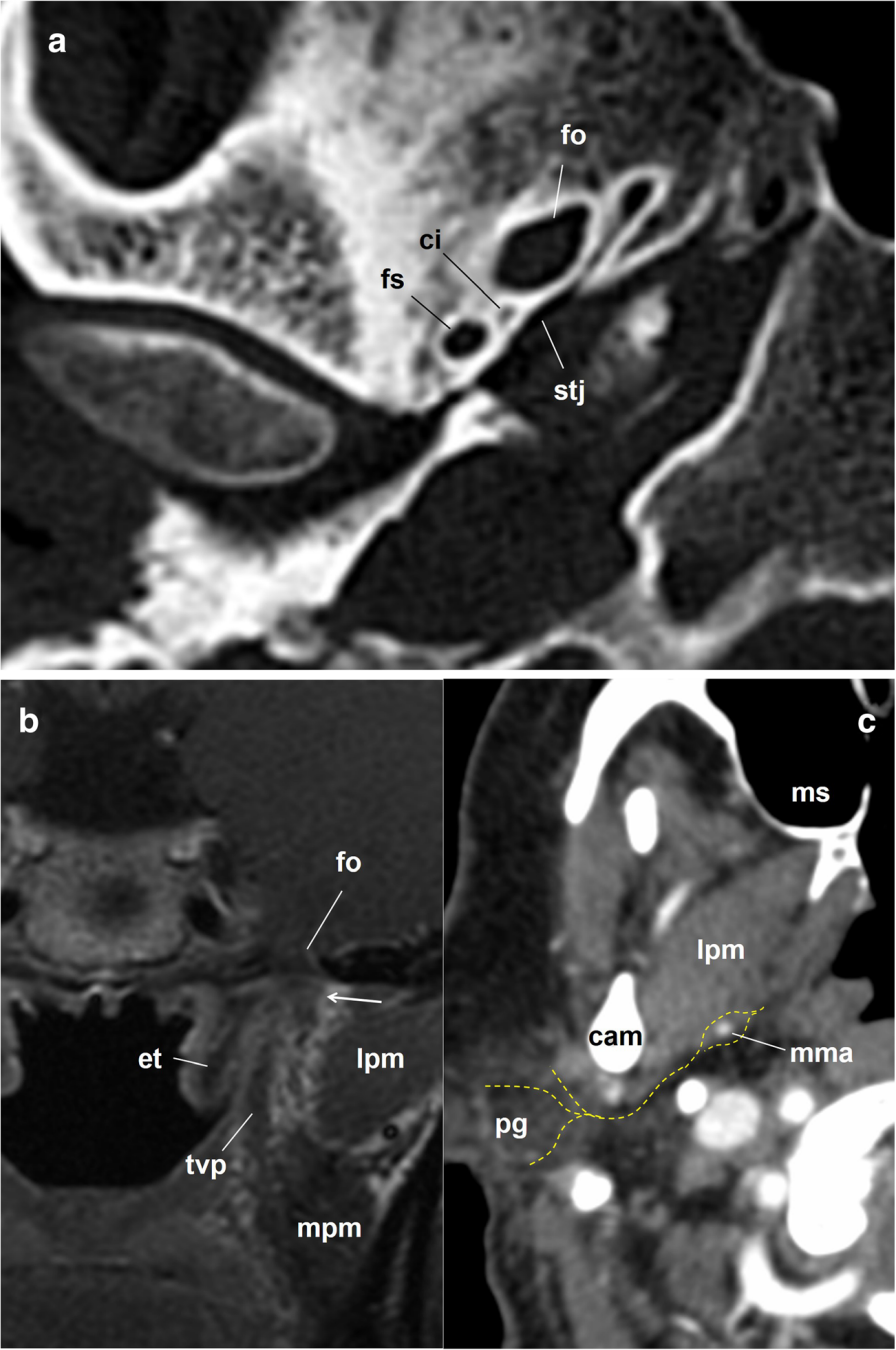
The glossopharyngeal nerve. a Skull base axial CT scan; right temporal bone, bone window. The image shows the foramen ovale, the foramen spinosum, the canaliculus innominatus (ci) of Arnold and the spheno-temporal junction, all of which are possible exit pathways of the lesser petrosal nerve. b Coronal fast spin-echo contrast-enhanced T1-weighted brain MRI, left side skull base. The images show the relationship between the foramen ovale (fo) and the V_3_ mandibular nerve and otic ganglion complex (arrow). et: Eustachian tube; lpm: lateral pterygoid muscle; mpm: medial pterygoid muscle; tvp: tensor veli palatini muscle. c Axial contrast-enhanced CT scan of the neck, soft tissues window. Glossopharyngeal nerve and auriculotemporal nerve. Just below the foramen ovale, the glossopharyngeal nerve fibres leave the otic ganglion to merge with the auriculotemporal nerve, a branch of the trigeminal nerve (the nerve pathway is represented by dashed yellow lines). Then, the nerve travels posteriorly in the parapharyngeal space to embrace the middle meningeal artery (mma), crosses along the medial edge of the condylar apophysis of the mandible (cam) and enters the parotid gland (pg). lpm: lateral pterygoid muscle; ms: maxillary sinus
